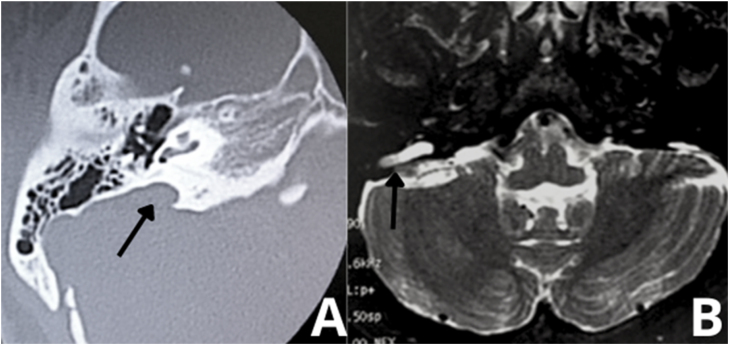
Enlargement of vestibular aqueduct (black arrows). (A) Right ear. Axial scan. Temporal bone high-resolution computed tomography. (B) MRI ‒ T2-weighted sequence of the same patient.
